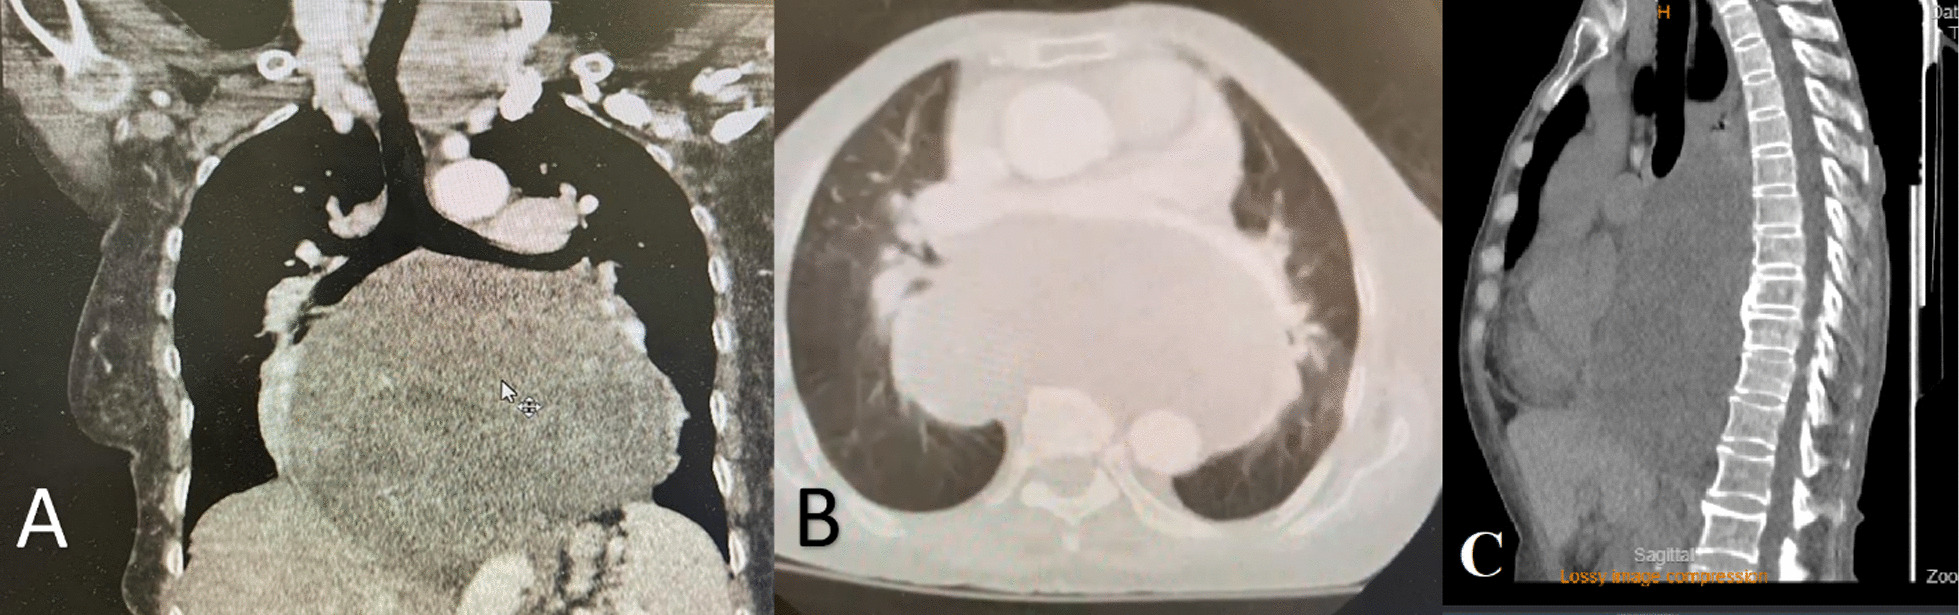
Chest computed tomography (CT) scan showing a large, hypodense, well-circumscribed posterior mediastinal mass (160*80 mm); A coronal view; B axial view; C Sagittal view
